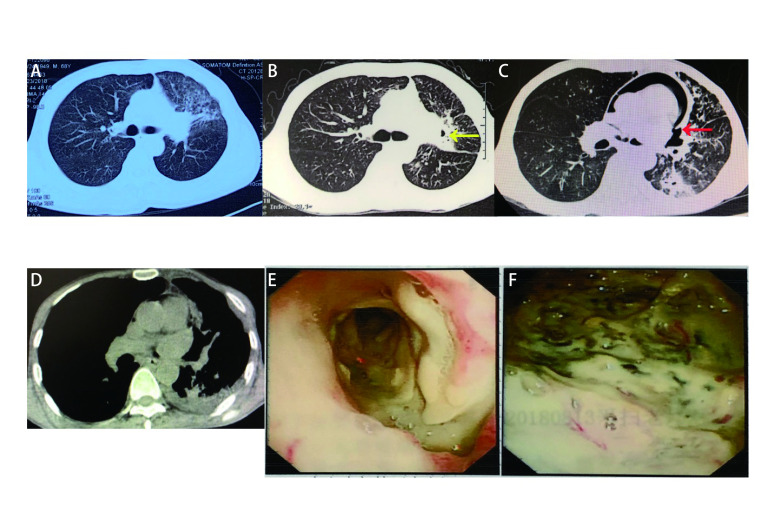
Broncho-pericardial fistula (BPCF) appears in a patient with pulmonary squamous cancer after using anlotinib. A: Left hilar mass (28 mm×31 mm), before use of anlotinib; B: After use of anlotinib for 1.5 mon, a cavity was formed in the left upper lung tumor (yellow arrow: cavity); C: A fistula communicating with the left main bronchus and pericardial cavity with large gas buildup in it, suggesting BPCF (red arrow); D: After drained gas with catheter stetted into pericardium for 5 days, computed tomography showed significant reduction of gas and pericardium partially conglutinated; E: (Bronchoscopy) A huge fistula at the left main bronchus; F: (Bronchoscopy) A huge cavity was seen with heart beat visible on the inner wall.
